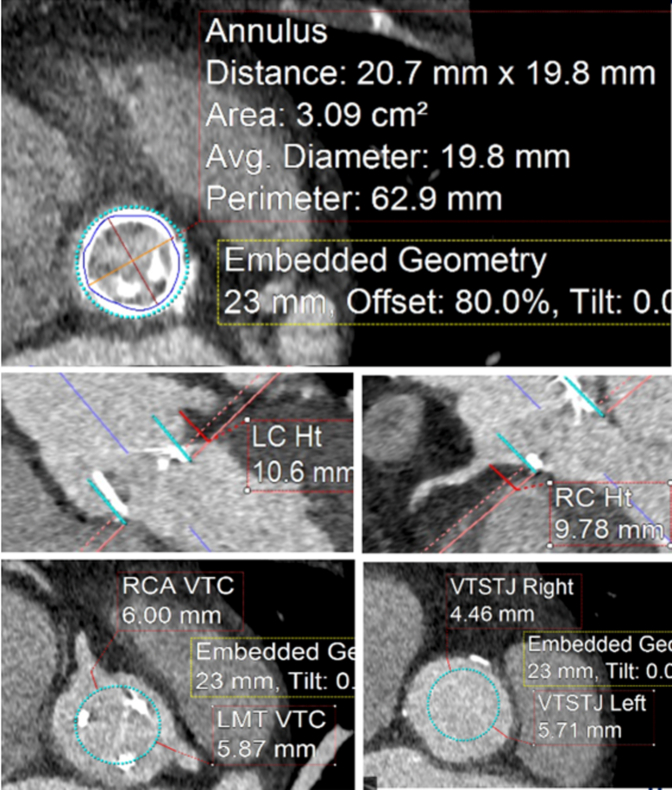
Transcatheter Aortic Valve Replacement Protocol Computed Tomography Angiography Images With Planning Data for Valve-in-Valve Transcatheter Aortic Valve Replacement The RCA height was <10 mm (9.78), indicating increased risk of CO. RCA VTc and VTstj were 0.6.00 mm and 4.46, respectively. Also shown are left main trunk height (10.6 mm), VTc (5.87 mm), and VTstj (5.71 mm). CTA = computed tomography angiography; LC = left coronary; LMT = left main trunk; RC = right coronary; RCA = right coronary artery; VTc = virtual transcatheter heart valve-to-coronary distance; VTstj = virtual transcatheter heart valve-to-sinotubular junction distance.
